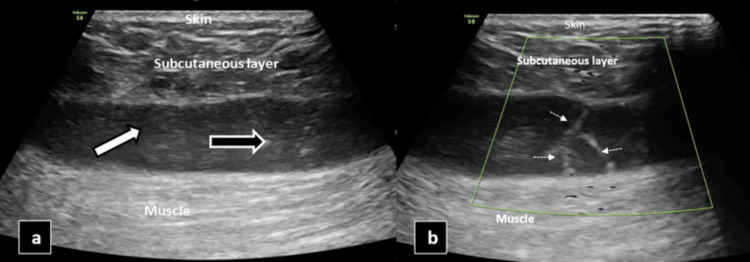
USG superficial image of the right thigh (a) showing anechoic fluid collection between the subcutaneous layer and muscle by the white arrow and a few internal echoes likely fat lobules represented by the black arrow. USG color Doppler (b) shows multiple tiny linear hyperechoic internal septations with no evidence of internal vascularity likely avascular septae represented by white dotted arrows.
